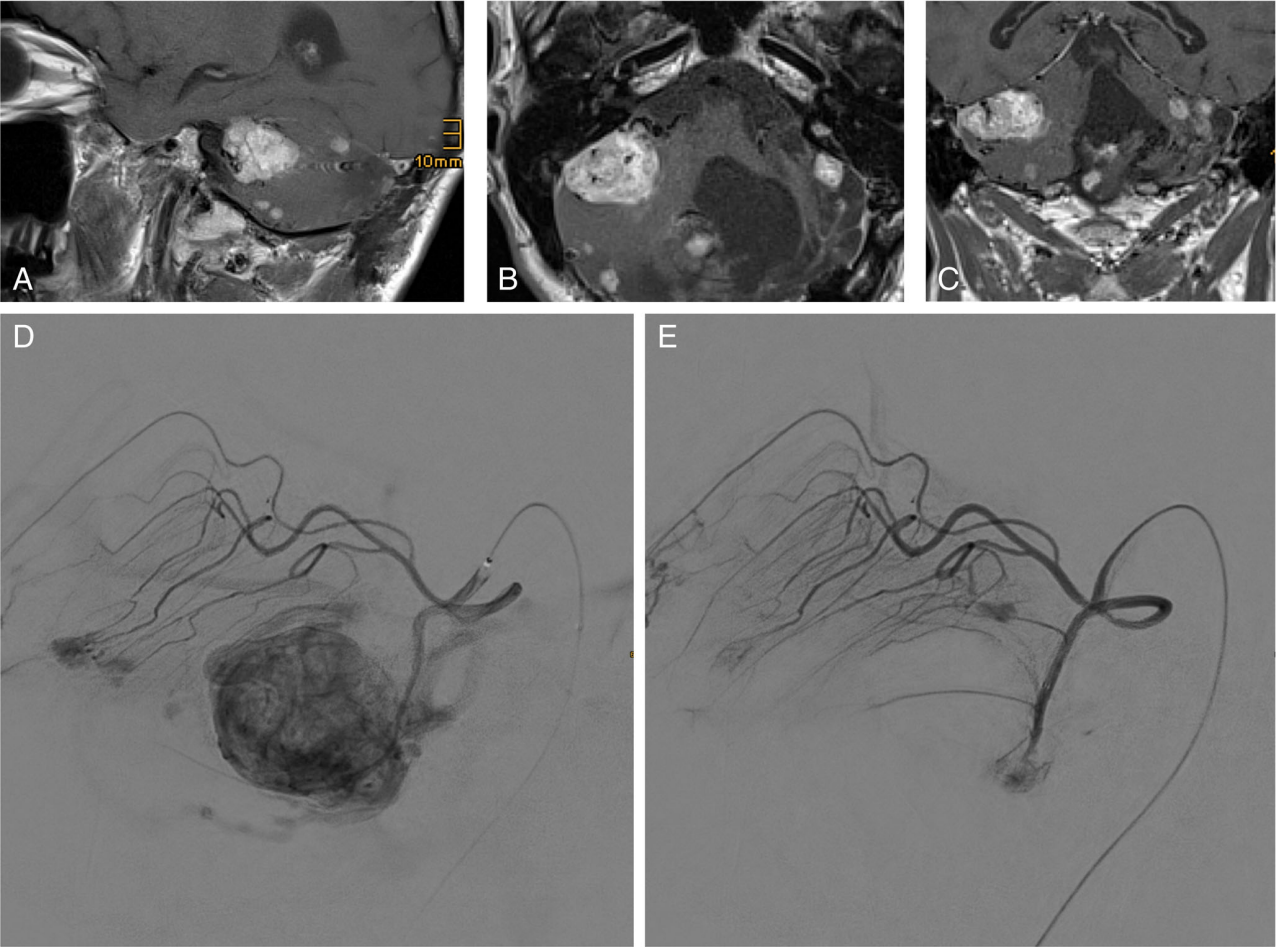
Preoperative embolization. Triplanar contrast-enhanced, T1-weighted MRI scan (a, b, c) predominantly showing a large solid tumor of the right posterior fossa in a VHL patient. Notice the large pathological blood vessels in and around the tumor (black) (b). Preoperative angiography (d) depicting a large tumor blush of the hemangioblastoma that is mainly nourished from the superior cerebellar artery. After particle embolization (e), the blood supply has been completely eliminated
